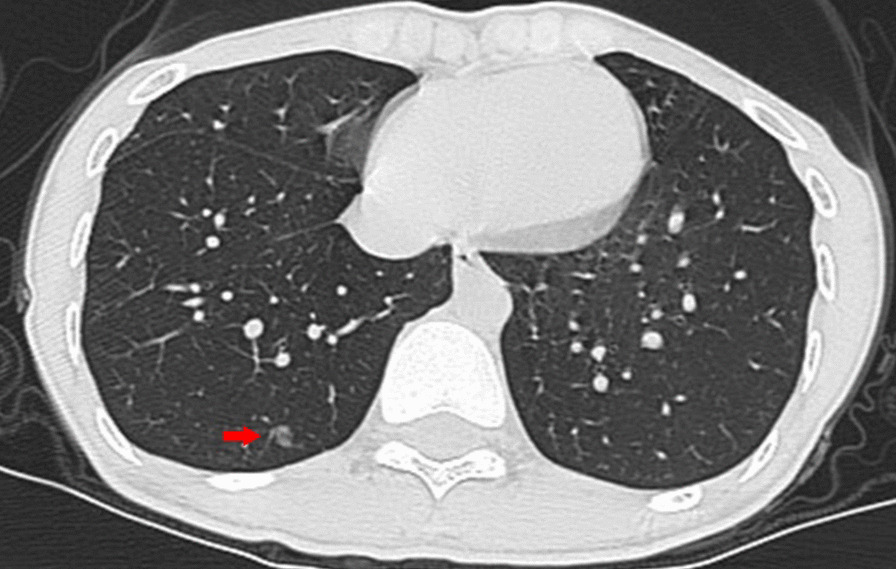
Plain chest CT image shows a ground glass opacity nodule (red arrow) in the right lower lobe of the lung
